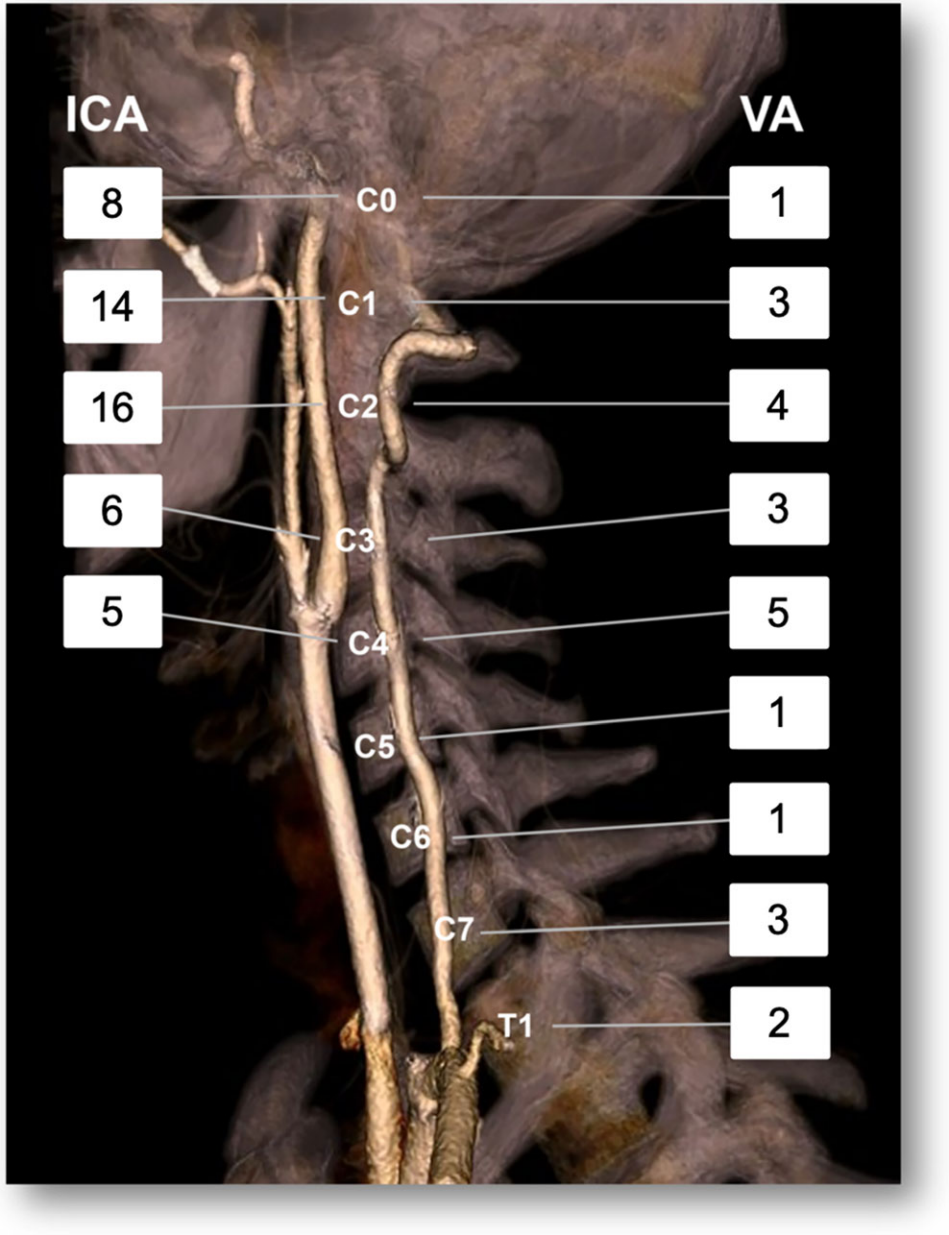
Number of BCVI by vertebral level in both ICA and VA on a surface-rendered CTA image without acute pathology
